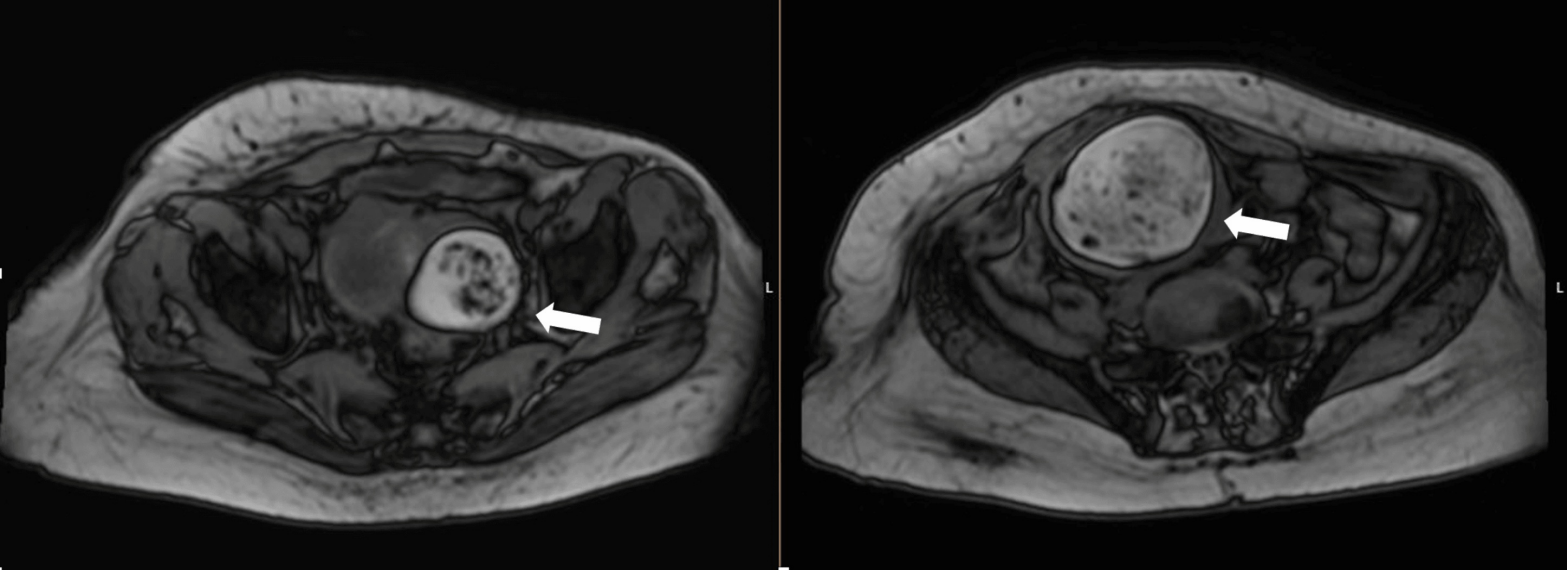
Axial MRI scan of the pelvis showing bilateral adnexal masses (white arrows) with predominant fatty components.
